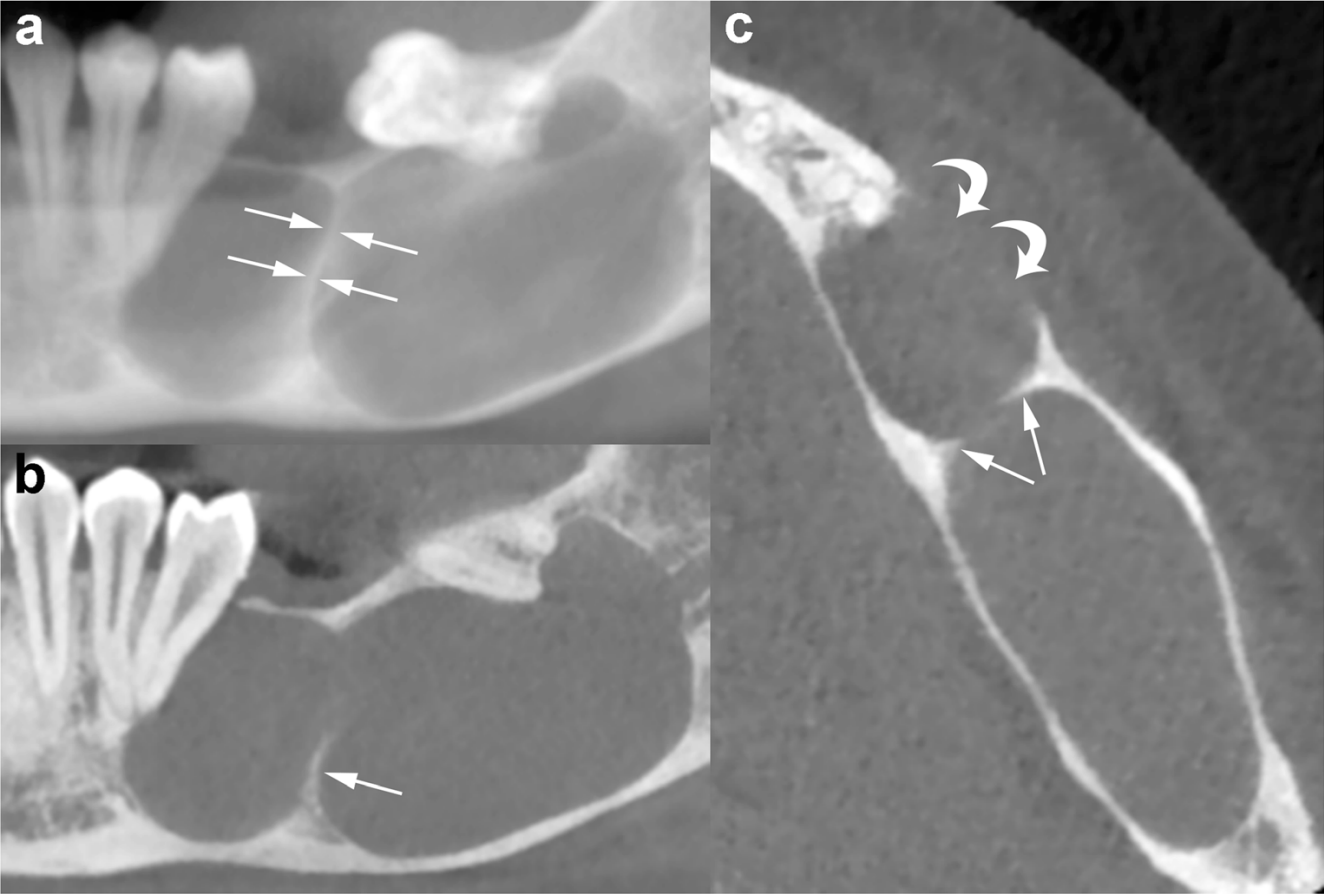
Panoramic CBCT image with 20-mm slice thickness (a) shows a mandibular OKC with a septum (*opposing arrows*) which seems to divide the lesion into two large loculations. Note the displacement of adjacent teeth. Panoramic (b) and axial (c) CBCT images reconstructed as 0.5- and 0.2-mm-thick sections demonstrate that the septum is incomplete (*arrows*). Perforation of the buccal cortex in the anterior portion of the lesion is also shown (*curved arrows*)
